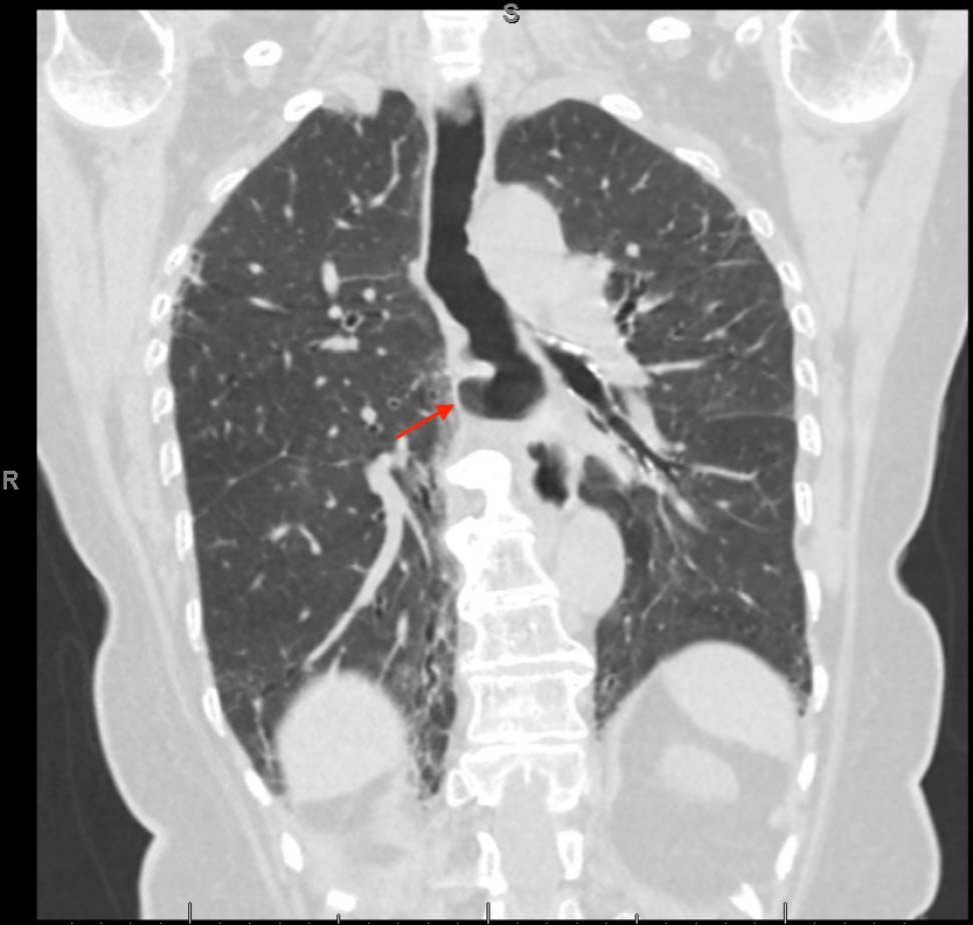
Computed tomography scan 2 months before presentation showing esophageal diverticulum (red arrow).
